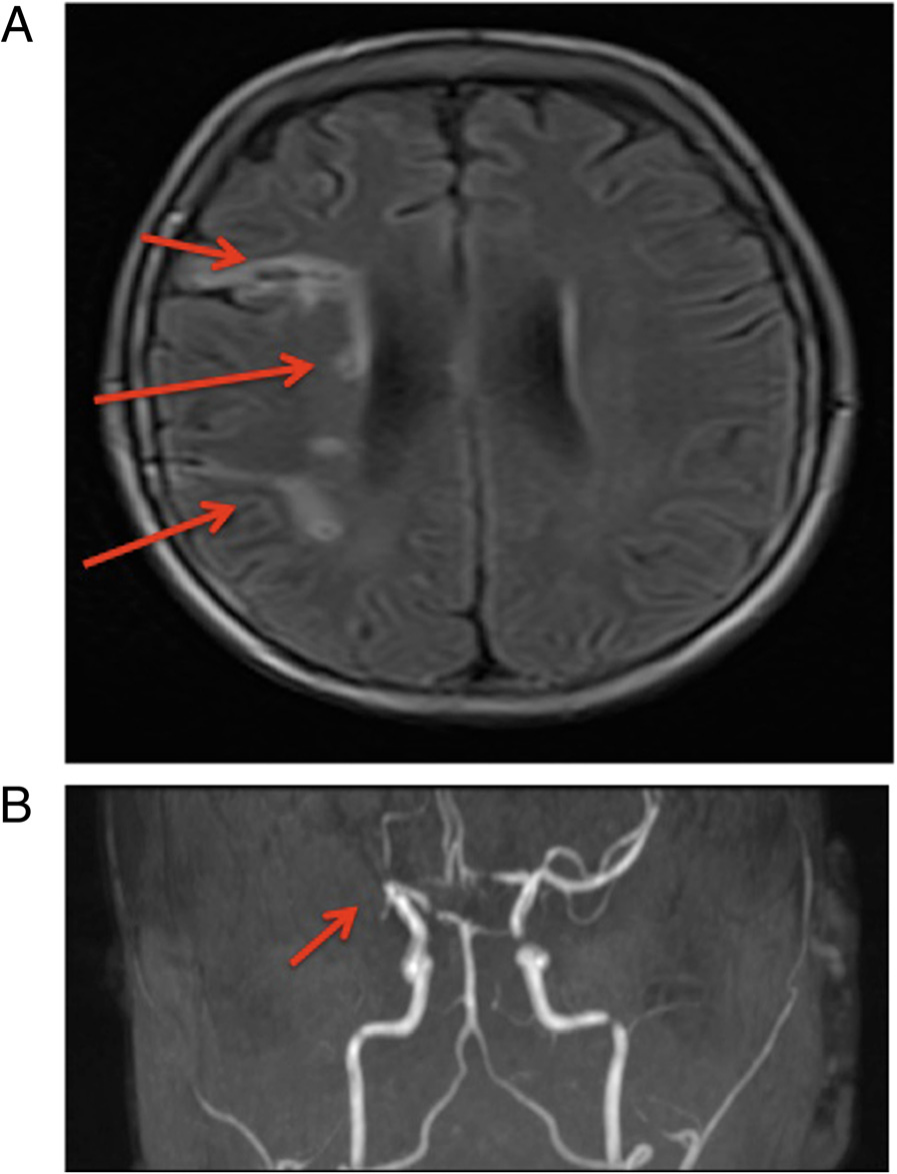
Cranial MR Imaging of the cerebral lesions. (A) Initial cranial MRI demonstrating right-sided temporo-parietal and thalamic lesions of different age. (B) MR angiography depicting missing flow signal of the right middle cerebral artery.
